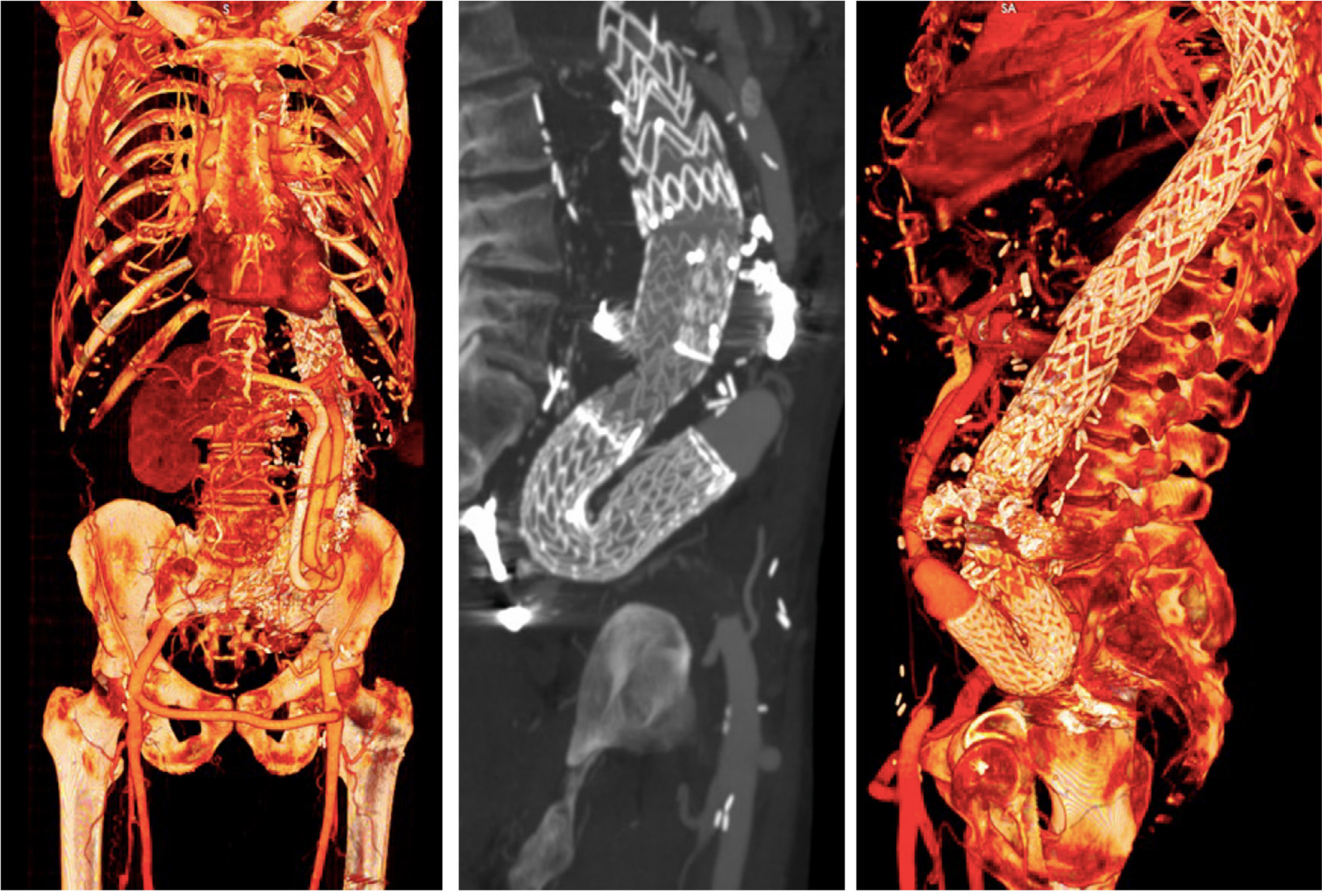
Computed tomography reconstructions showing endograft placement, with a 180° curved shape within the visceral debranching Dacron graft without kinking or endoleaks.
